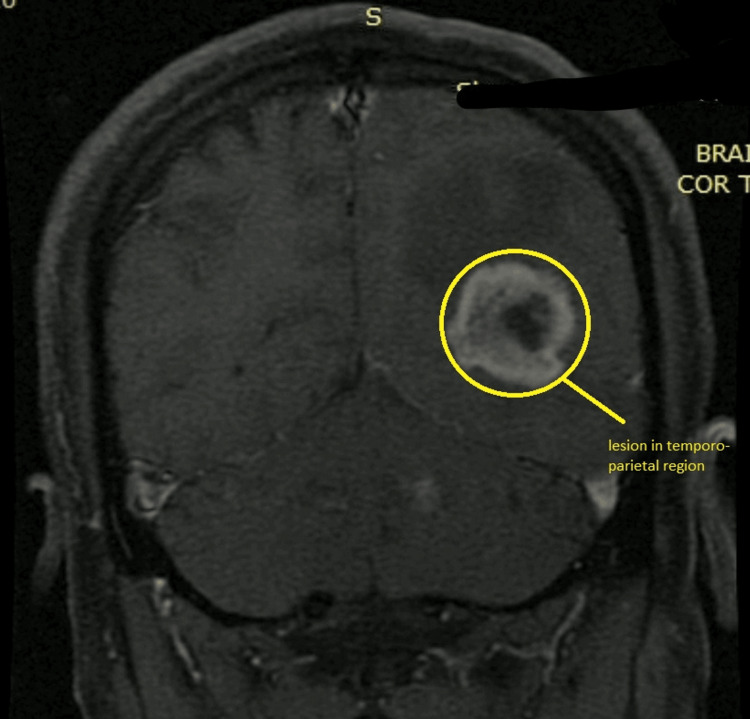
Coronal view of the MRI showing lesion in the left temporoparietal region
